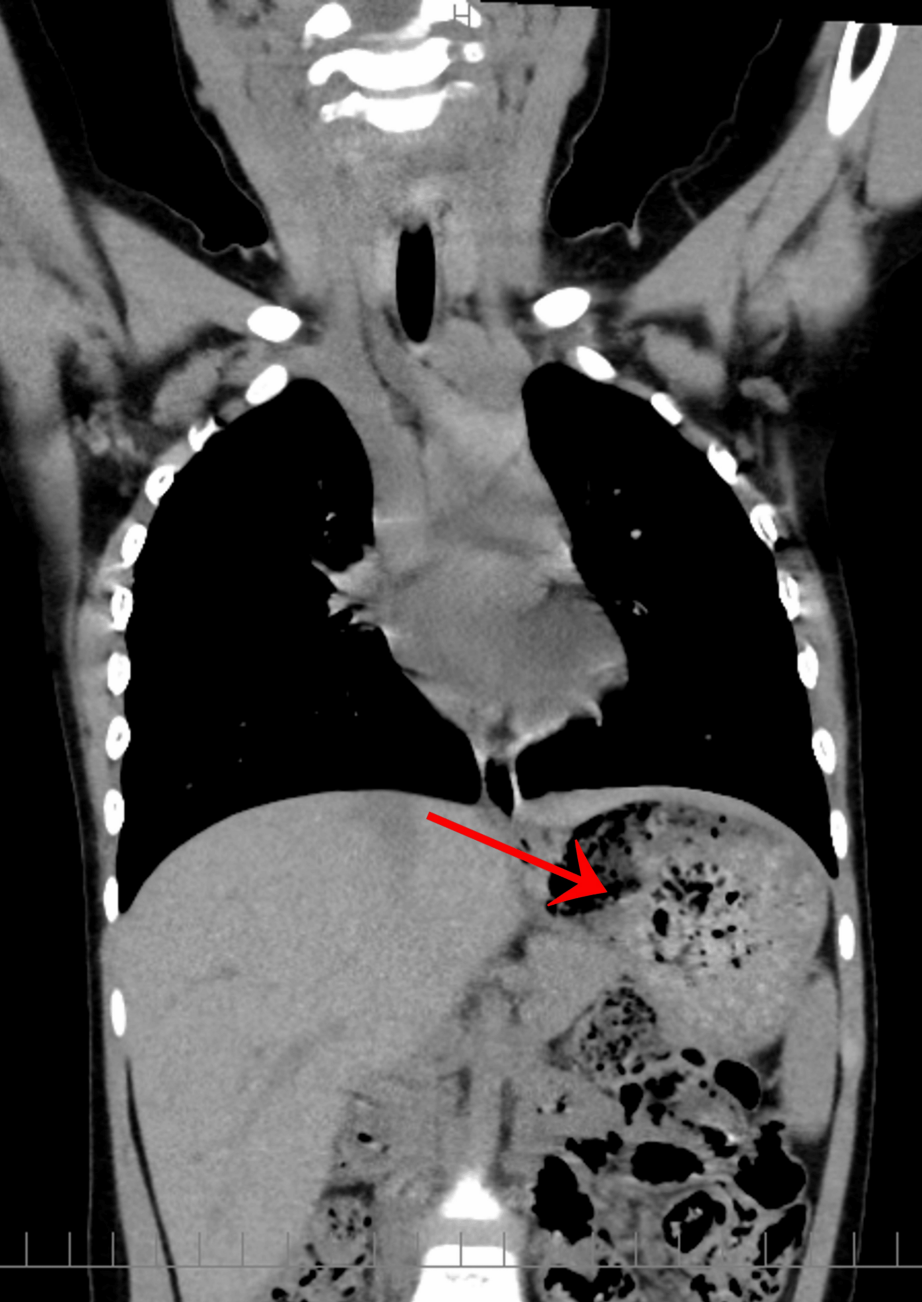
A CT scan from the neck to the abdomen There was a large amount of residual food in the stomach, but no foreign body could be identified.
